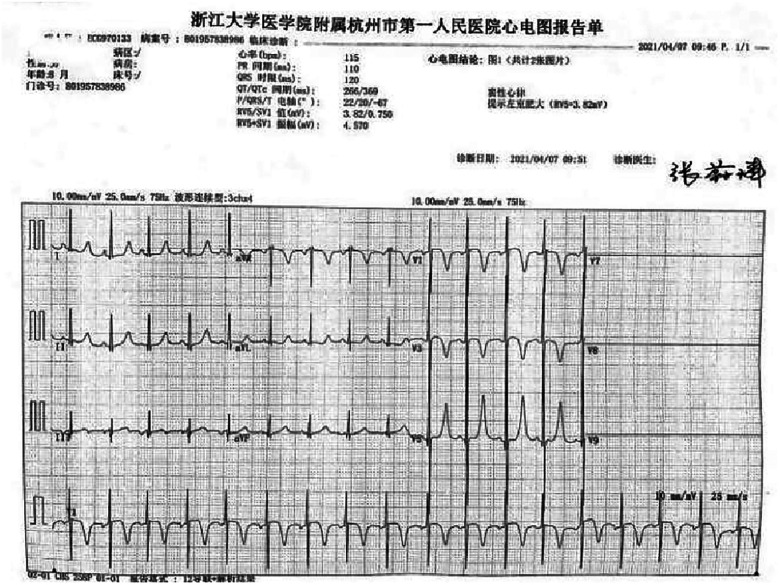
Echocardiography at 32 m.
